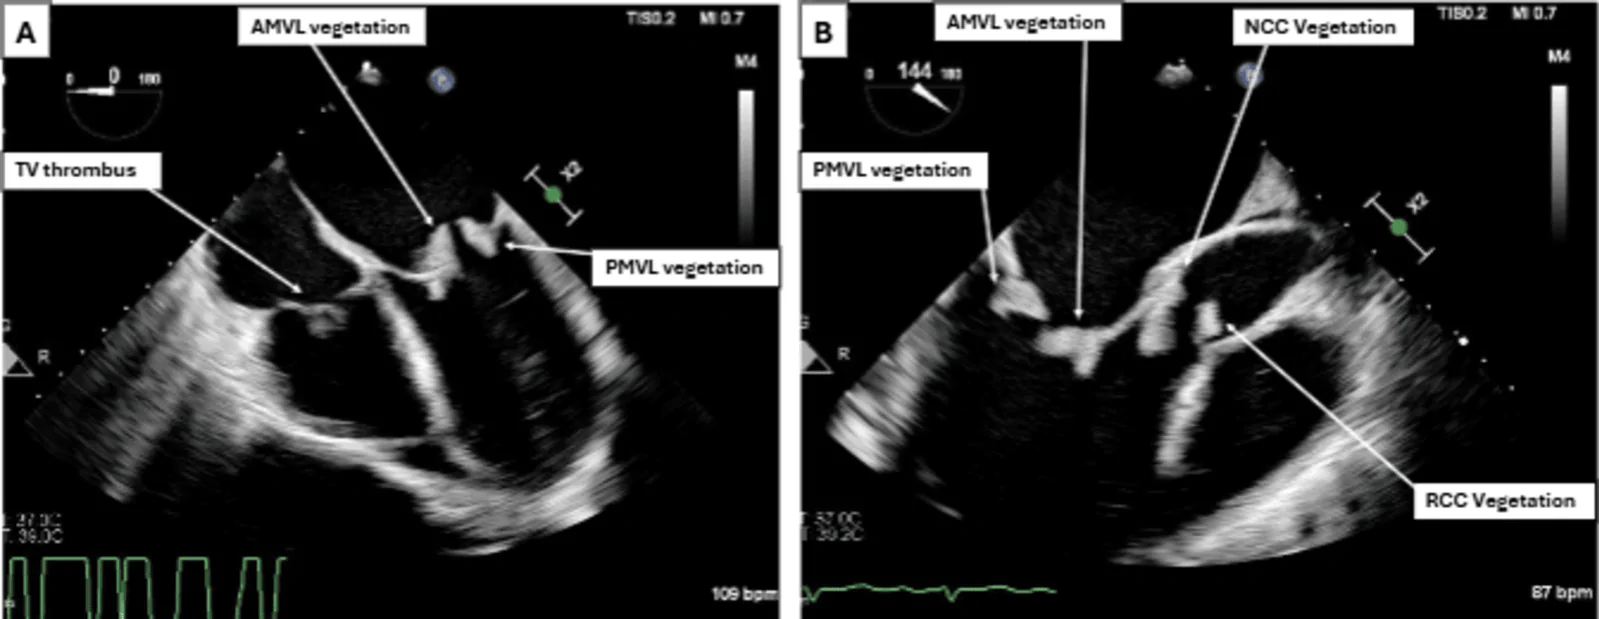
TEE images depicting valvular endocarditis (A) Mid-esophageal A4CH TEE view showing vegetations on the AMVL and PMVL, as well as masses attached to the TV with different echogenicity from the left-sided valvular vegetations, more consistent with thrombotic material. (B) Mid-esophageal long-axis TEE view showing vegetations on both the AMVL and PMVL and on the RCC and NCC of the aortic valve. A4CH, apical four-chamber; AMVL, anterior mitral valve leaflet; NCC, noncoronary cusp; PMVL, posterior mitral valve leaflet; RCC, right coronary cusp; TEE, transesophageal echocardiography; TV, tricuspid valve
